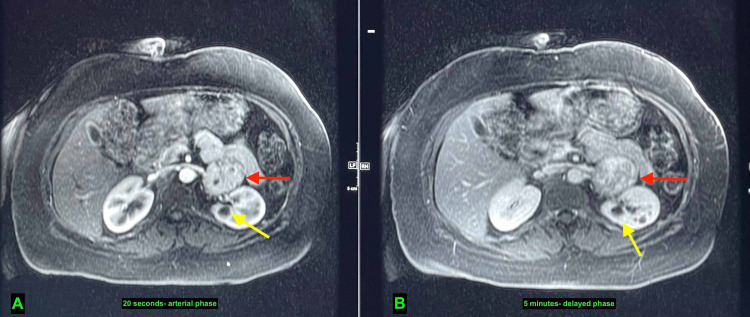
Axial view- T1-weighted post contrast MRI. A, early enhancement of left adrenal mass in arterial phase (red arrow), and renal cyst (yellow arrow). B, persistent enhancement of left adrenal mass in delayed phase (red arrow), and renal cyst (yellow arrow). MRI: magnetic resonance imaging
